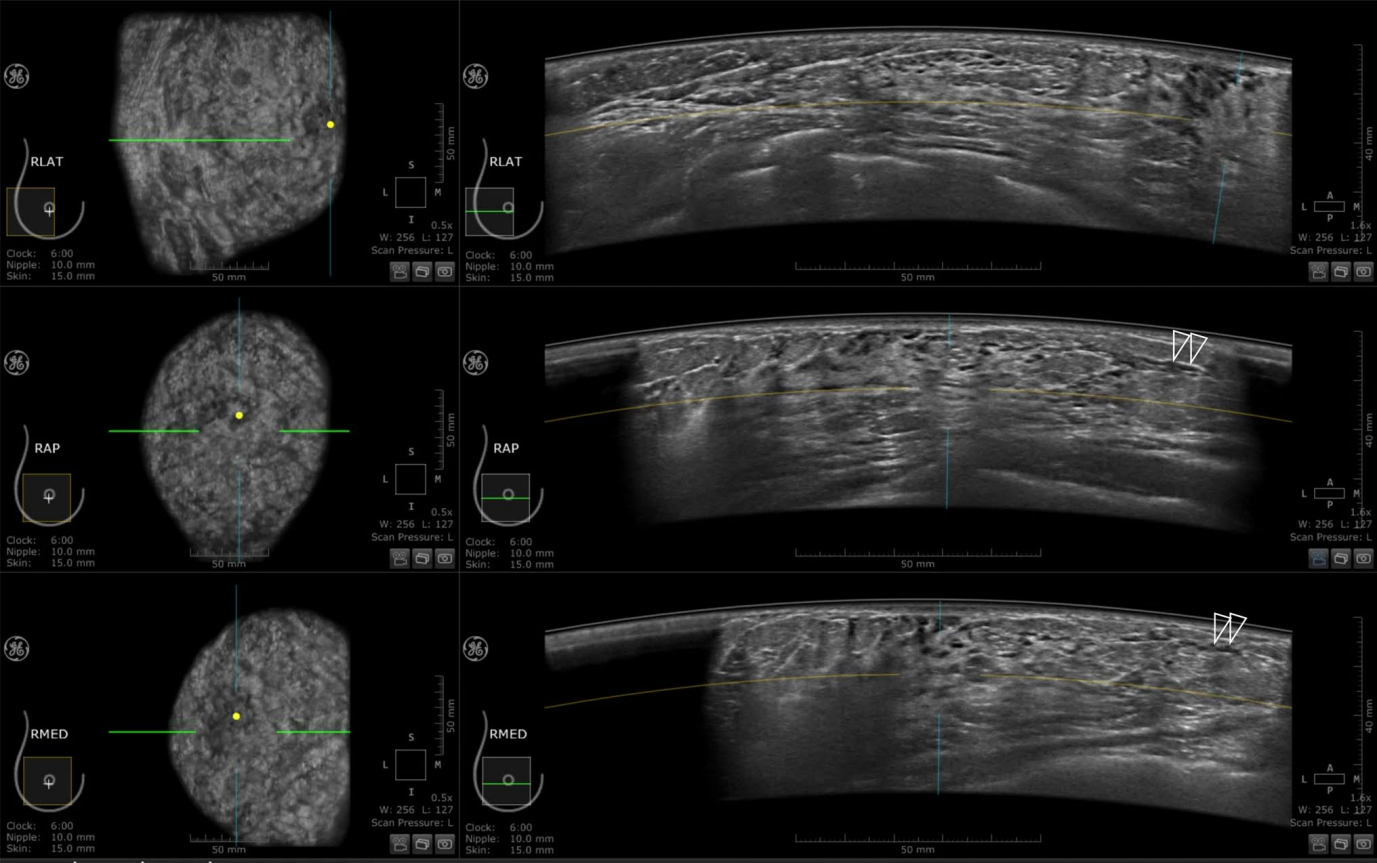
Right breast ABUS images of a 29-year-old woman at 20 weeks 5 days of pregnancy. Lateral (LAT), anteroposterior (AP), and medial (MED) images from top to bottom, with a coronal section presented on the left and a horizontal section on the right. These sonograms show ducts extending to the margins of the breast where little echogenic fibroglandular tissue could be seen (double open arrowheads)
